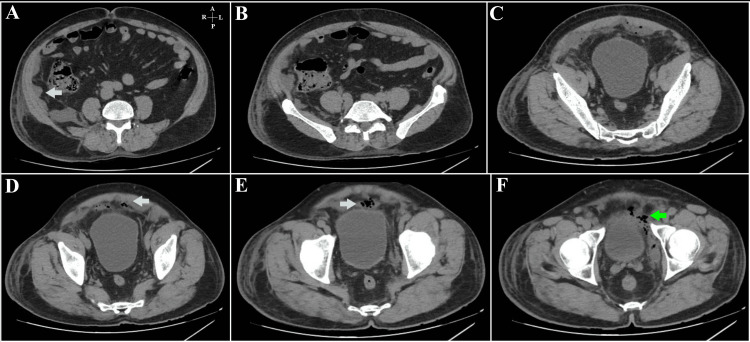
Patient 5- CT images of the abdomen and pelvis in the axial plane Figure 7A– Through the L3 vertebral body; a fluid density collection (cyan arrow) is seen in the extra-peritoneal space on the right with peritoneal thickening and stranding of fat. Figure 7B– Extra-peritoneal fat stranding is noted on the right, with a collection in the extra-peritoneal space posterior to the ascending colon. Figure 7C– An air-containing collection is noted in the pre-vesical and right anterior abdominal wall with peritoneal thickening and extensive regional fat stranding. Figure 7D– Axial image of the pelvis with an air-containing collection (cyan arrow) seen posterior to the rectus abdominis muscle, anterior to the urinary bladder. Figure 7E– A loculated collection with multiple air foci (cyan arrow) is seen anterior to the urinary bladder with fascial thickening and fat stranding. Figure 7F– At the level of the femoral heads; a heterogeneous air-containing collection (green arrow) is noted in the pre-vesical and left hemi-pelvis, medial to the anterior aspect of the obturator internus muscle.
